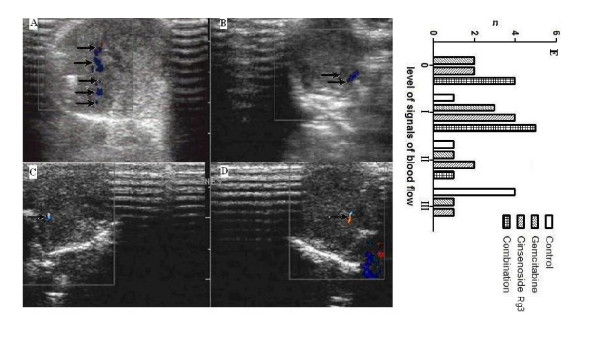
Observation of blood flow in tumors by color Doppler flow imaging (CDFI). A: more than five dot-like blood flow signals and three club-like blood flow signals (arrow) detected in tumors in the control group; B: dot- and club-like blood flow (arrow) in tumors in the gemcitabine group; C: a short-like blood flow (arrow) in tumors in the ginsenoside Rg3 group; D: a thin- and short-like blood flow (arrow) in tumors in the combination group. E: levels of signals of blood flow. Signals of blood flow in tumors of CDFI were classified into four grades: 0, no blood flow signals detected within the tumor; I, minimal blood flow (one or two dot-like or a thin- and short-like blood flow signals detected within the tumor); II, moderate blood flow (up to three dot-like blood flow signals or one longer blood flow signals detected within the tumor); and III, abundant blood flow (more than five dot-like blood flow signals or two longer blood flow signals detected within the tumor).
